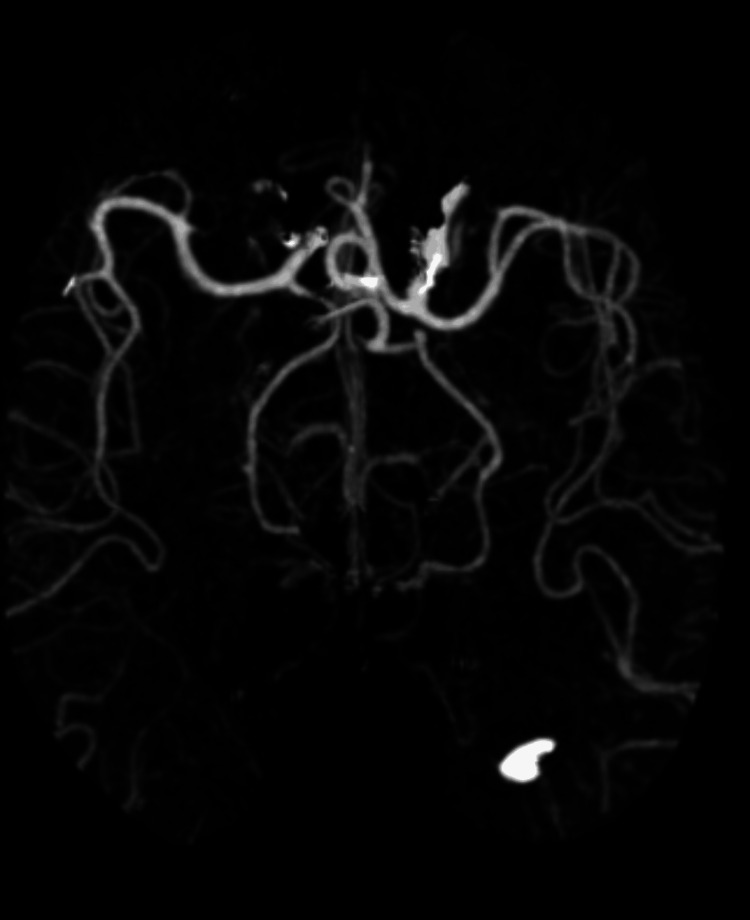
CT angiography of head and neck which demonstrated no significant stenosis or occlusion of major arteries.
